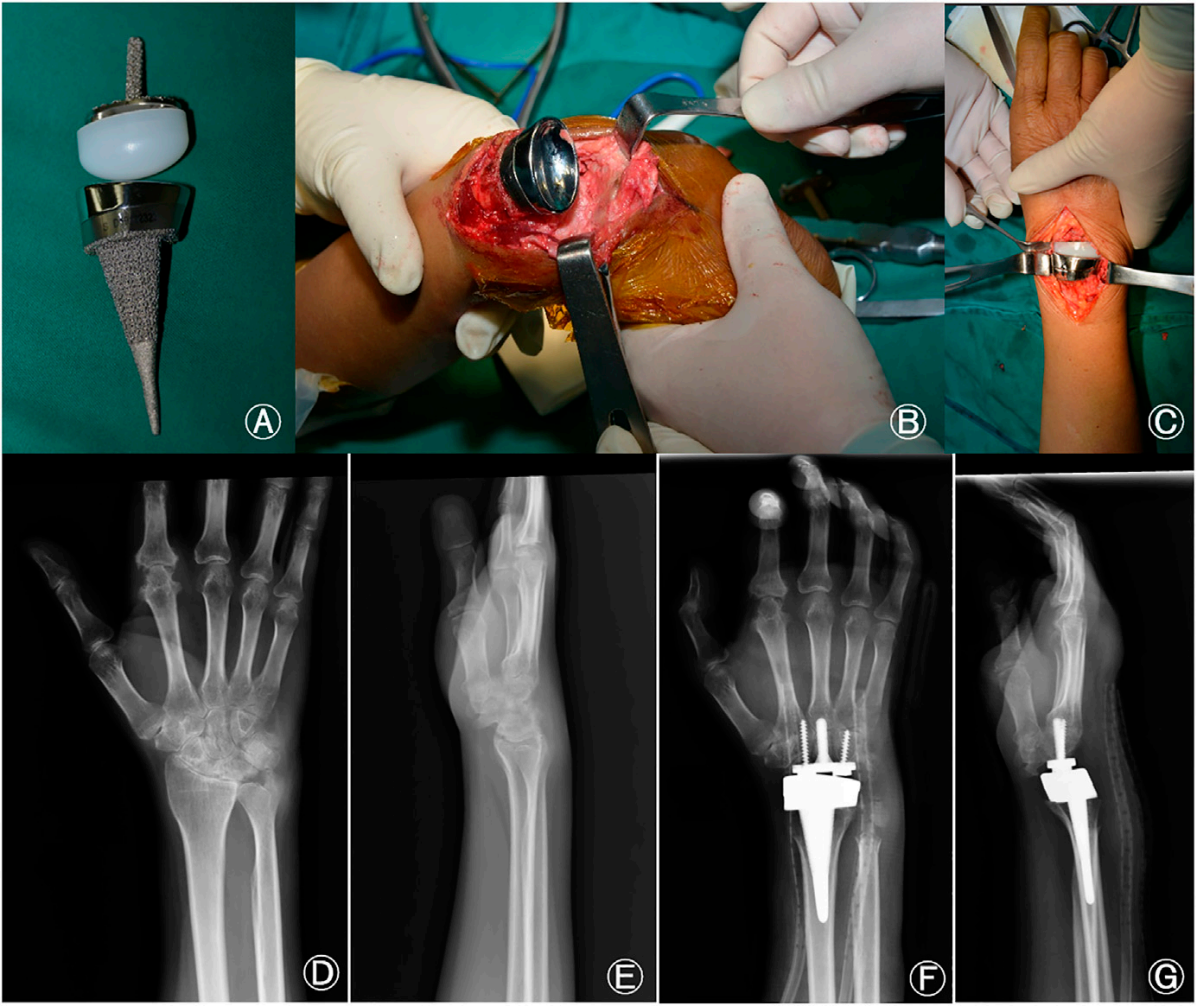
(A) Microporous titanium total wrist joint prosthesis, (B) Intraoperative carpal and radial osteotomy have been completed and the radial component is being installed. (C) Polyethylene has been inserted into the carpal component and the artificial wrist joint has been reset, (D) Preoperative posteroanterior radiographs of the wrist, (E) Preoperative lateral radiographs of the wrist, (F) Posteroanterior radiographs of the wrist at 1 month after TWA, (G) Lateral radiographs at 1 month after TWA. TWA, total wrist arthroplasty.
